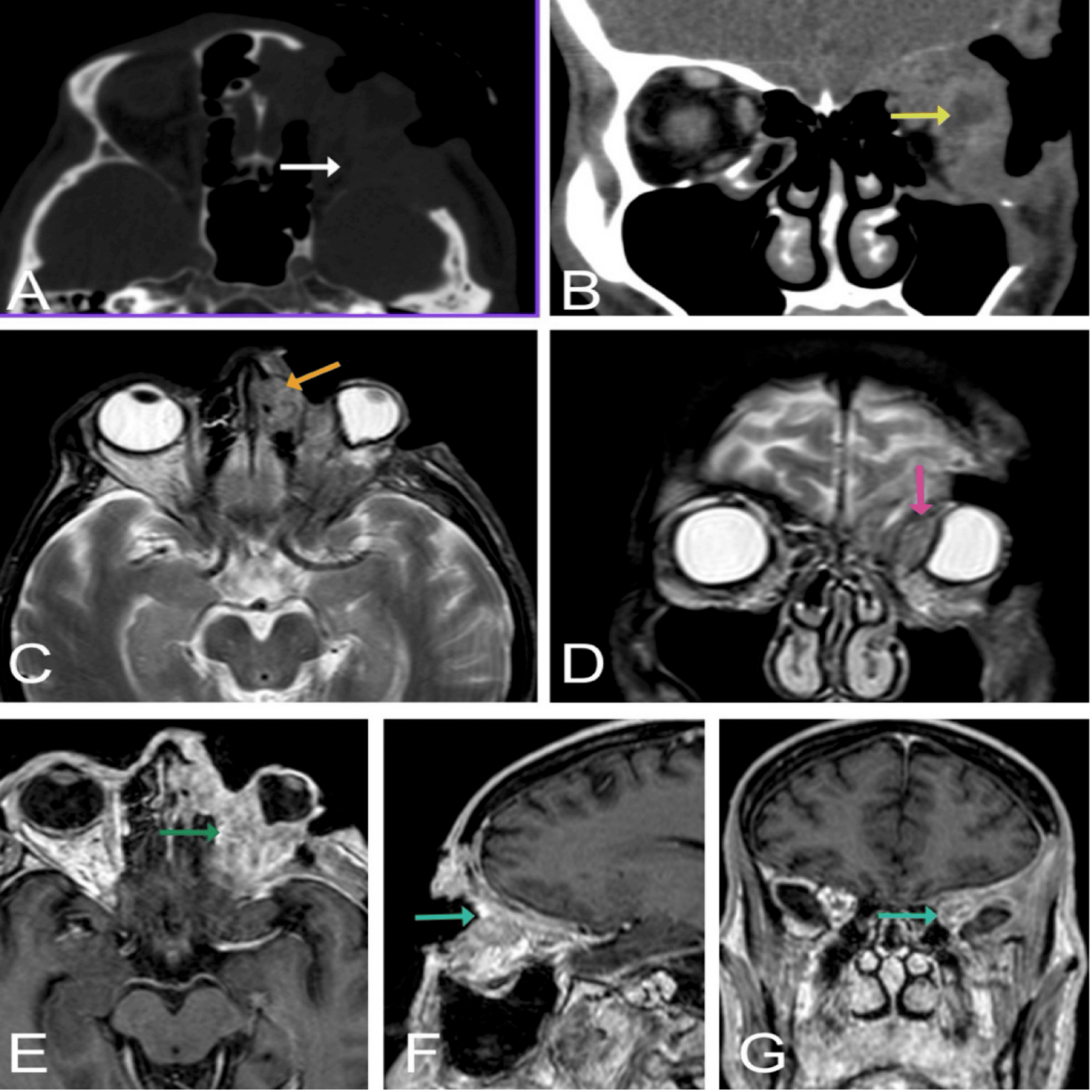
Orbital and facial computerized tomography (CT) and magnetic resonance imaging (MRI) Axial bone window (A) and coronal soft tissue window (B) in contrast-enhanced CT demonstrate a heterogeneously enhancing infiltrative soft tissue left orbital lesion causing mass effect on the left eye globe (yellow arrow), which appears deformed and compressed. There is additional infiltration of the extraocular muscles and osseous destruction/erosion of the left orbit, left maxillary sinus, left lamina papyracea and anterior left ethmoid air cells, left zygoma, left frontotemporal skull, and left sphenoid wing (white arrow). T2-weighted axial (C) and coronal (D) MRI demonstrate locally invasive heterogeneous T2 left orbital mass with obliteration of the extraconal fat, extension into the left anterior ethmoid air cells (orange arrow), and infiltration of the extraocular muscles with marked asymmetric enlargement of the left medial rectus muscle (pink arrow). Axial (E), sagittal (F), and coronal (G) post-contrast T1-weighted 3D MRI sequence demonstrate diffusely enhancing irregular aggressive appearing left orbital mass (green arrow) with osseous extension/infiltration as evidenced by abnormal enhancement of the left orbital walls including the roof and orbital apex (blue arrows), sphenoid bone, through the lamina papyracea into the ethmoid air cells, and left zygoma.  There was no evidence of intracranial extension, parenchymal involvement, or abnormal intracranial post-contrast enhancement.
